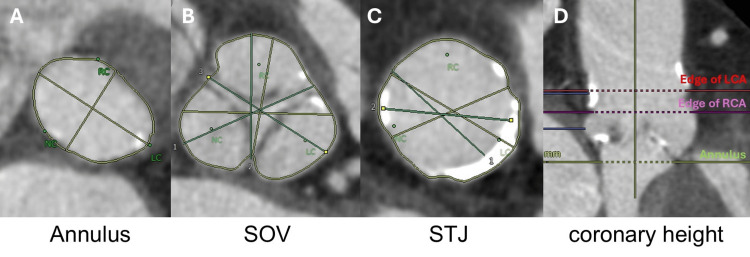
Preoperative CT of the patient’s aortic root LCA: left coronary artery; RCA: right coronary artery; SOV: sinus of Valsalva; STJ: sinotubular junction. A: Annulus level short-axis view. The annulus area was 319 mm^2^, and the valve circumference was 66 mm. B: SOV level short-axis view. The SOV sizes were 27, 28, and 30 mm. C: STJ level short-axis view. The STJ diameter was 23 x 24 mm and was highly calcified. D: Long axis view of aortic root. The LCA height was 19.7 mm, and the RCA height was 13.7 mm. The structure of the aortic valve base was adapted to the 26-mm Evolut pro + device.
